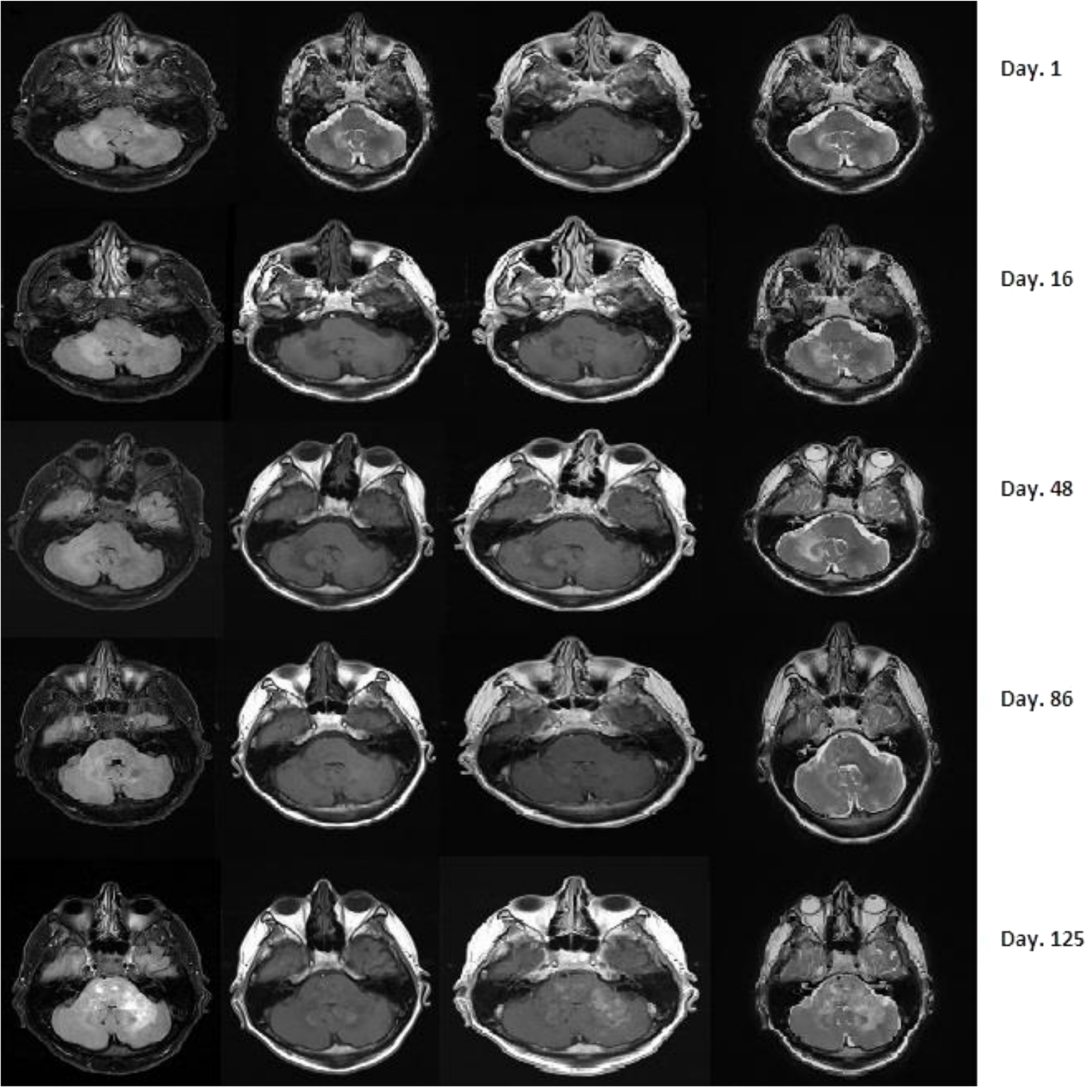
Showing MRI brain axial sequences FLAIR/T2/T1 and T1 with contrast at various stages. Day 1, new non-enhancing cerebellar hemisphere T2 hyperintensities (larger on the right). The new ill-defined extensive T2 hyperintensity in the right middle cerebellar peduncle extending into the right cerebellar white matter is felt concerning for PML. Day 16, no pathological enhancement identified. The previously noted new high signal in the right cerebellar peduncle extending into the right cerebellar hemisphere is slightly more extensive than on the prior scan but no enhancement. Day 48, progression of right cerebellar and brainstem high signal and enhancement. Progression of the presumed PML in the right middle cerebellar peduncle extending into the right cerebellar hemisphere and now extending to the right side of the pons. Day 86, area of abnormal T2 high signal within the right middle cerebellar peduncle extending into the pons and right cerebellum is again demonstrated. There is increased T2 signal at the periphery of the lesion within the right cerebellar hemisphere, and involvement of the pons is also significantly increased, now extending to involve the central pons. New area of T2 low signal within the medial left cerebellar hemisphere and in the left cerebellum adjacent to the inferior left cerebellar peduncle faint peripheral enhancement in the area of increased FLAIR signal in the right cerebellar hemisphere. Faint enhancement within the peripheral aspect of the right cerebellar lesion suggestive of PML-IRIS. MRI findings are consistent with progression of disease with progressive involvement of the pons and new involvement of the left cerebellar hemisphere. Day 125, significant progression of high signal abnormality in the posterior fossa as described, now extending to the left of the midline associated with extensive patchy enhancement. The appearances would now be more in keeping with PML-IRIS. (These MRI-images are acquired through 1.5 T Machine)
